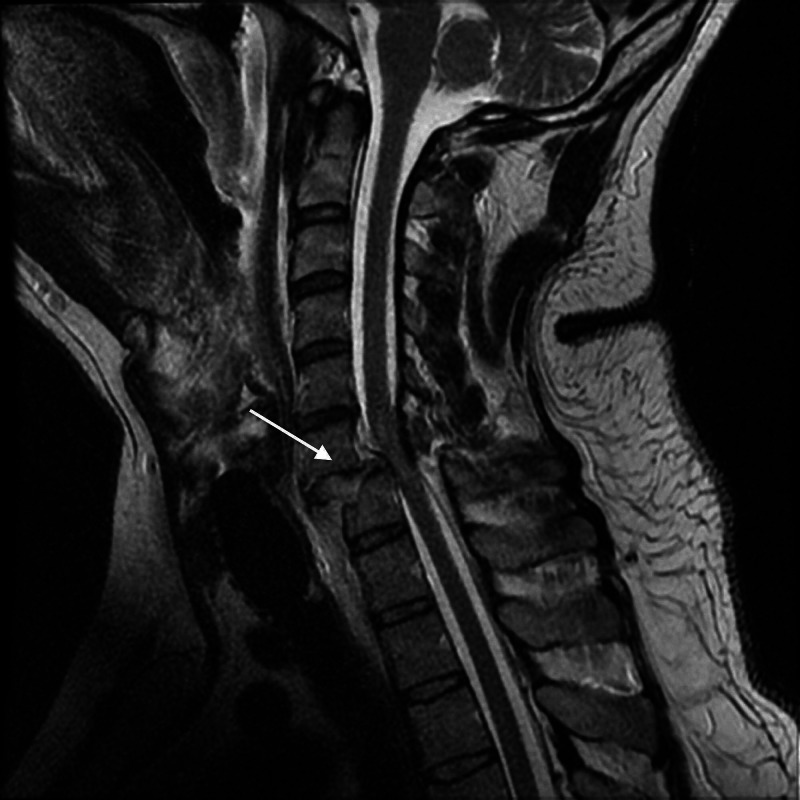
T2 sagittal cuts of an MRI (the same case as in Figure 2) demonstrating a complete rupture of the ligamentum flavum at C6-7 with related short-segment posterior epidural hematoma, and disrupted ALL at C7 with pre-vertebral hematoma, also showing the extent of damage to the intervertebral disc ALL, anterior longitudinal ligament Case courtesy of Dr Andrew Dixon, https://radiopaedia.org/, rID: 31837
